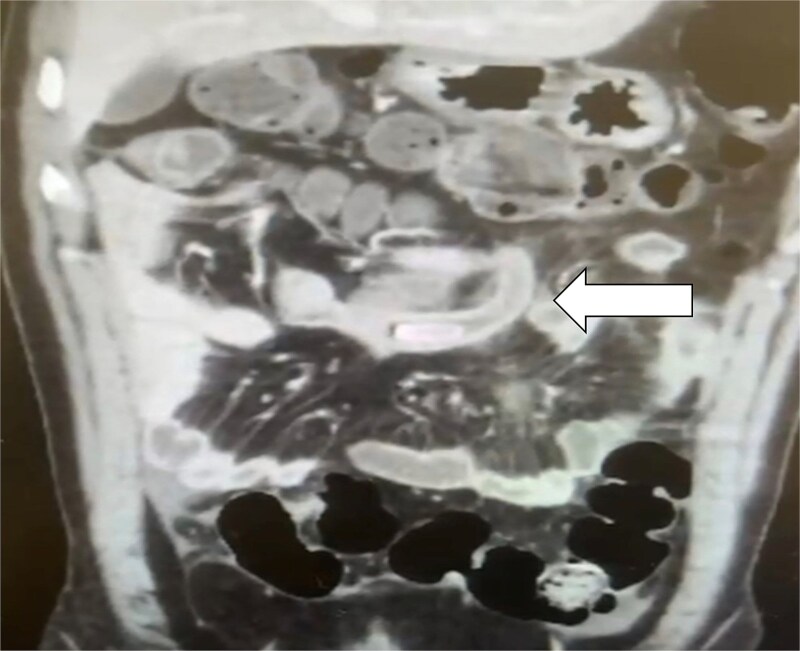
CT scan coronal view demonstrating the appendix with appendicolith at the mid-abdomen due to incomplete malrotation.
